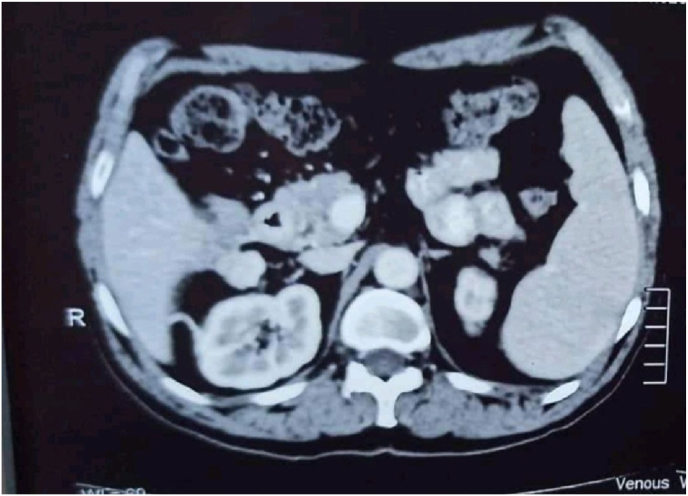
CT abdomen showing encapsulated mass arising from 2nd part of duodenum (axial section).
